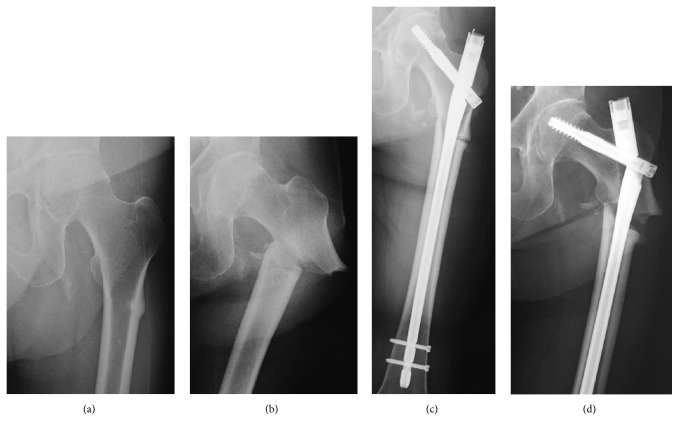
(a) An AP radiograph of the left femur shows a transverse fracture line and thickening of the lateral cortex at the subtrochanteric area. (b) A completely displaced fracture. (c) The internal fixation using a long femoral nail. (d) The nail breakage and opening of the fracture region.
